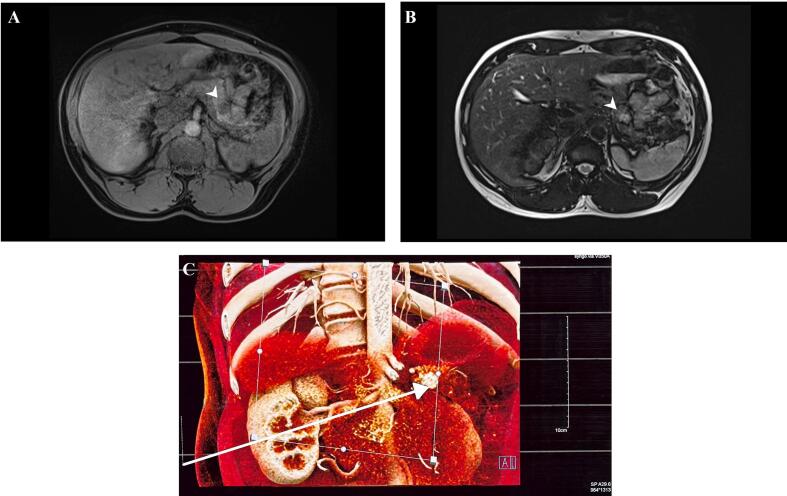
Abdominal MRI scan showing a pancreatic tumour (white arrowhead), (A) hypointense on T1 fat-suppressed sequence and (B) hyperintense on T2 fat-suppressed sequence – the classic imaging finding of pancreatic insulinomas. (C) 3D reconstruction of MRI sequences showing the insulinoma in the distal pancreas (white arrow).
